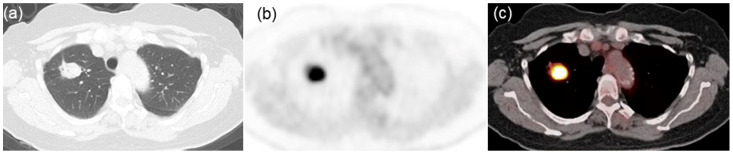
Representative images from a 69-year-old woman with lung adenocarcinoma characterized by low PD-L1 expression. (a) CT image displaying a lung mass in the right upper lobe. (b) FDG PET and (c) PET/CT fusion images exhibiting intense tracer uptake within the tumor (SUVmax = 11.56, LGRE = 0.0021, SRLGE = 0.0020, LRHGE = 685, LGZE = 0.0021, HGZE = 622, and SZLGE = 0.0017).
